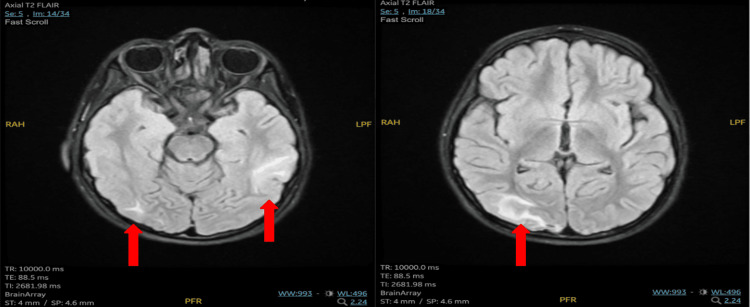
Axial T2 FLAIR MRI showing cortical/subcortical hyperintense signal in the right occipital and left temporo-occipital regions (arrows) MRI, magnetic resonance imaging; FLAIR, fluid-attenuated inversion recovery
